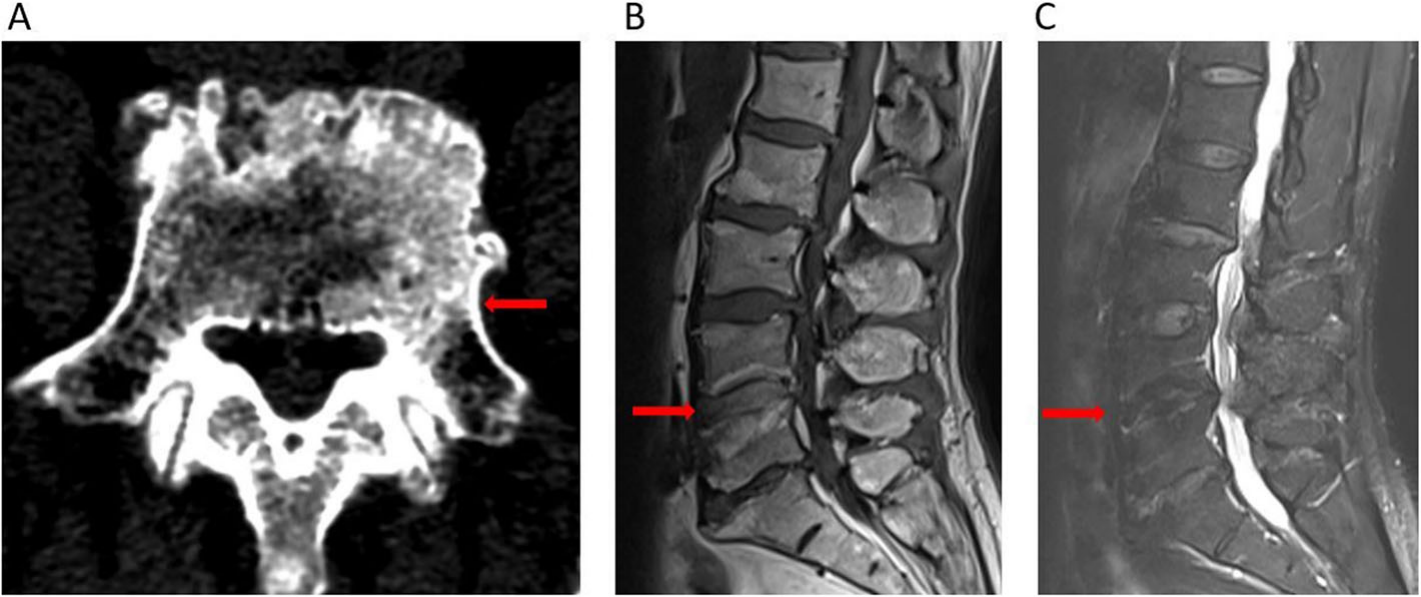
Radiological findings at the 36th month after treatment. Notes: (a) CT showed just hyperplasia and deterioration of L5 and no recurrence of vertebral destruction (arrow). (b and c) MRI showed there was no indication of inflammatory signal (hypointense on T1-WI and heterogeneous hyperintense on T2-WI) (arrow) compared with the images before
